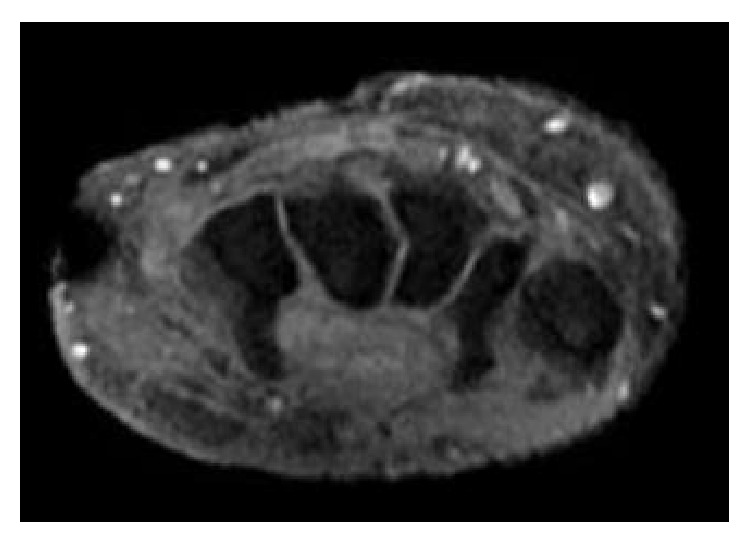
MRI of the left arm showed multiple ulcerations extending from skin into subcutaneous fat with appearance concern for necrotizing fasciitis and osteomyelitis.
